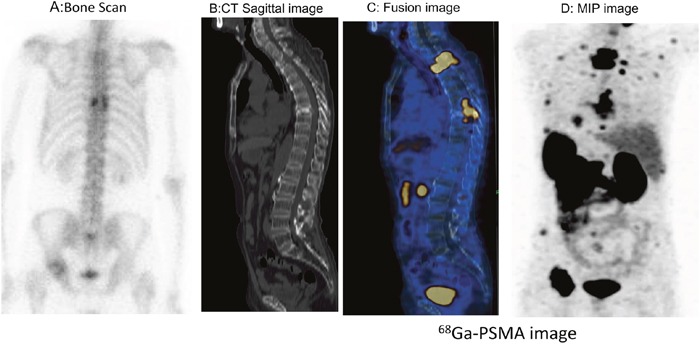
Thoracic spinal cord invasion was detected on 68Ga-PSMA-11 PET/CT images in a patient with mCRPC Multiple PSMA-avid lesions in the thoracic spine, ribs and pelvis were also detected. The spinal cord was also involved (SUV_max_ = 110). A. Bone scan. B. CT image. C, D. ^68^Ga-PSMA-11 PET /CT images: C, fusion image; D, MIP projection.
